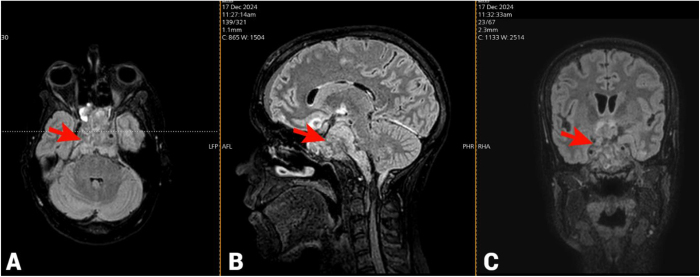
Brain MRI with T2W/FLAIR sequences of the brain demonstrating evidence of disease recurrence: (A) axial, (B) sagittal, and (C) coronal views. There is a large heterogeneous sellar and suprasellar mass with heterogeneously high signal intensity on T2W/FLAIR sequences. The mass appears invasive and extending in different directions. The sellar-suprasellar bulk measuring about 4.2 × 4.5 × 4.5 cm, posterior extension measures 1.5 cm in thickness (red arrows). MRI: magnetic resonance imaging.
